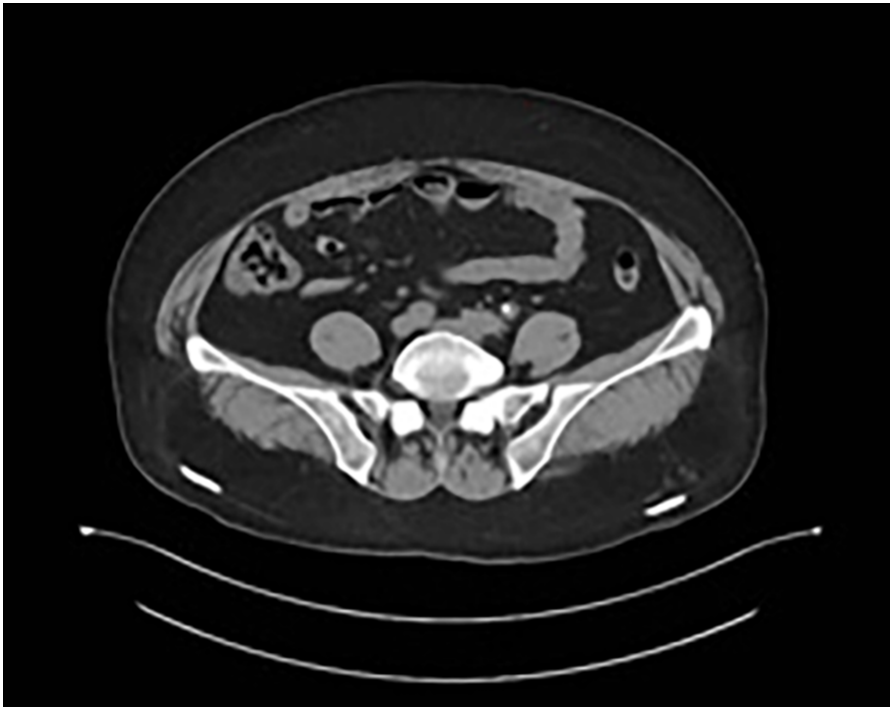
Follow-up CT after neoadjuvant chemotherapy demonstrating marked tumor regression (residual lesion: 0.7 × 0.6 cm) and normalization of ureteral caliber.
